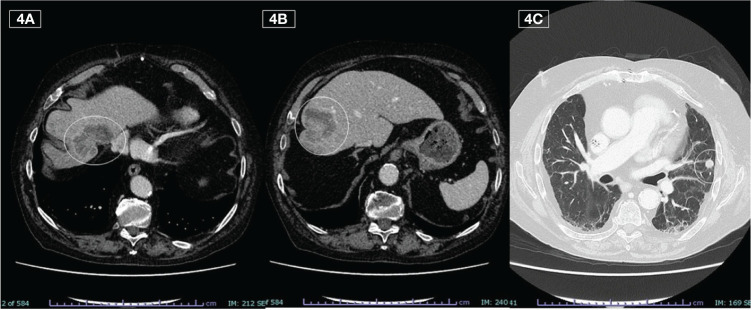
Liver (A, B) and lung (C) metastases detected on last CT during the off phase (September 2023)
